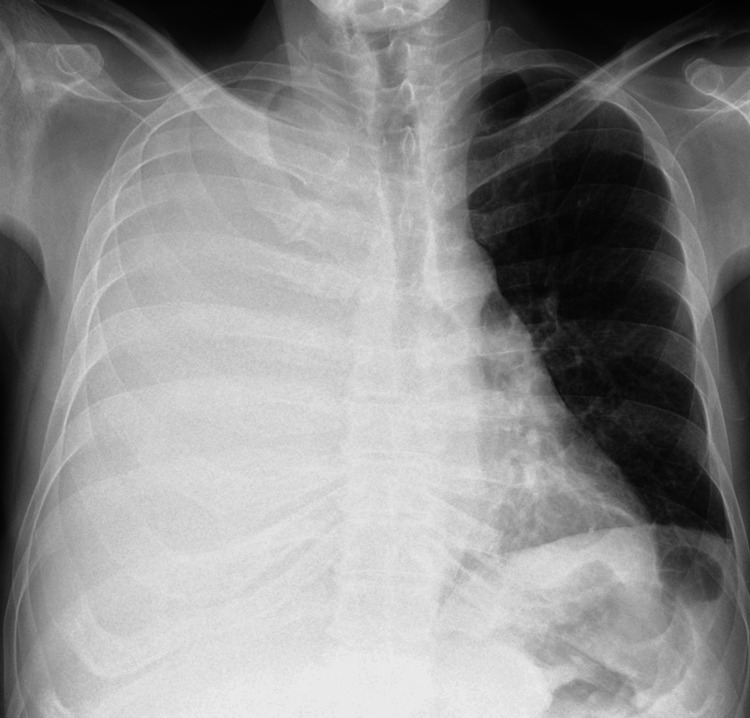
Chest radiography of the first patient revealing a large-volume right pleural effusion upon ICU admission
